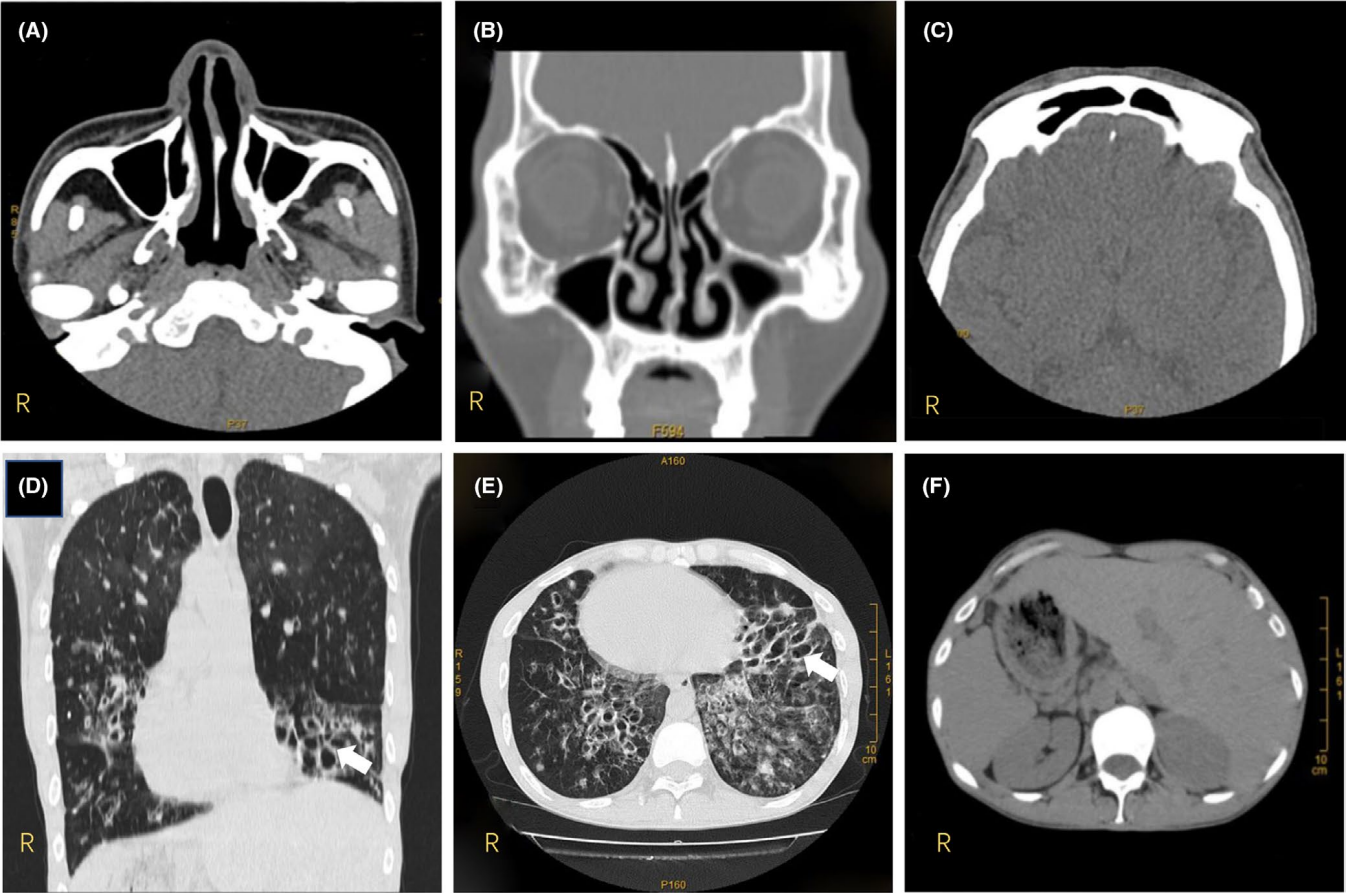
CT scan of the PCD proband (II:3). The paranasal sinus CT scan of the proband shows non‐specific thickening of the mucosa on the bilateral maxillary sinuses (A, B) and left frontal sinus (C). The chest CT scan shows multiple patchy high‐density shadows in bilateral lungs with blurred boundaries, bronchial dilatation, thickened bilateral bronchial walls (D, E) and *situs inversus* (D, E, F). Bronchiectasis is indicated by white arrows (D, E). CT, computed tomography; PCD, primary ciliary dyskinesia
